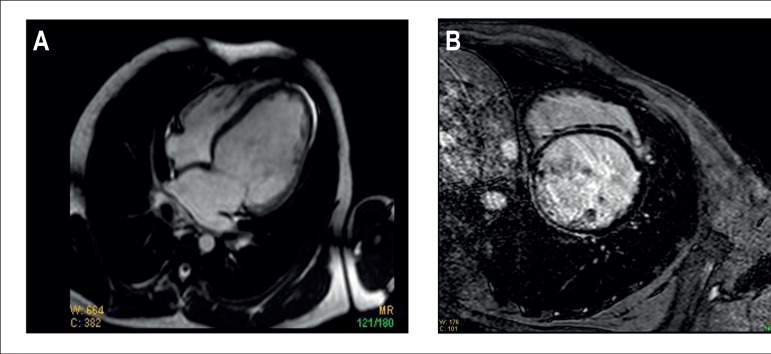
A) Cardiac MRI. Dilated left ventricle and atrium, presence of pericardial
